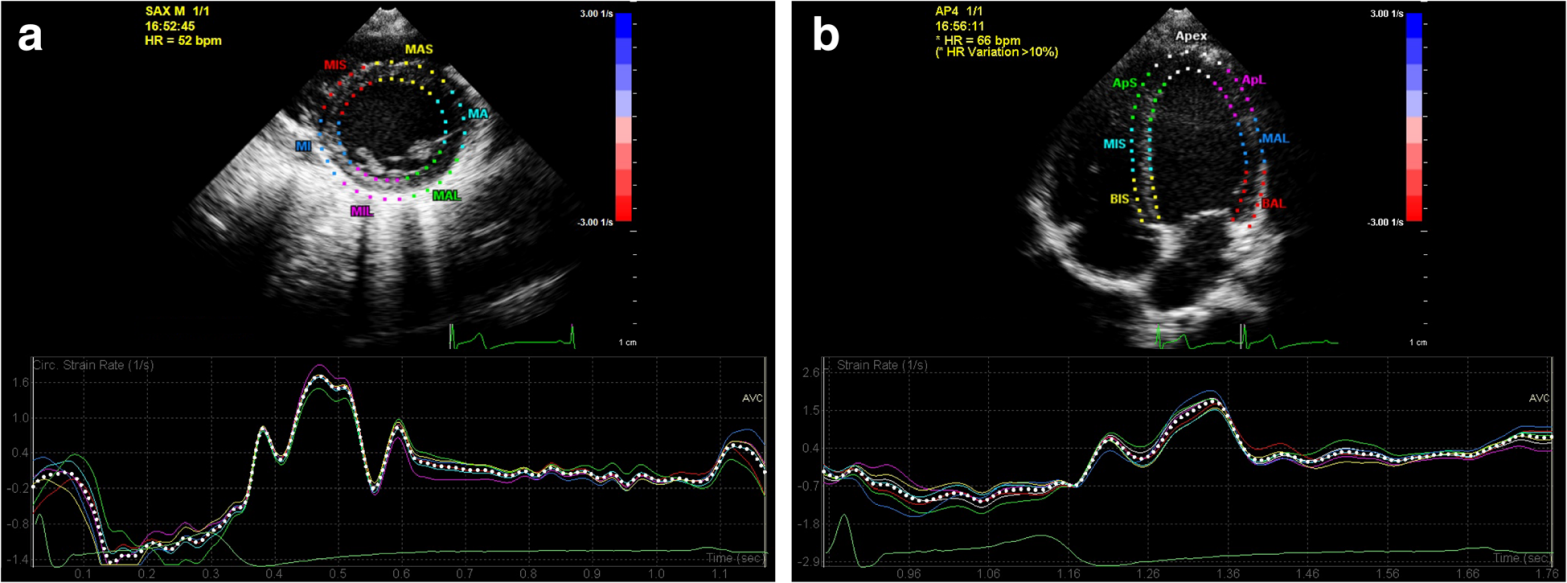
Echocardiographic image examples of speckle tracking derived left ventricular circumferential (a) and longitudinal (b) strain rate analyses in a patient with neurocardiogenic syncope
